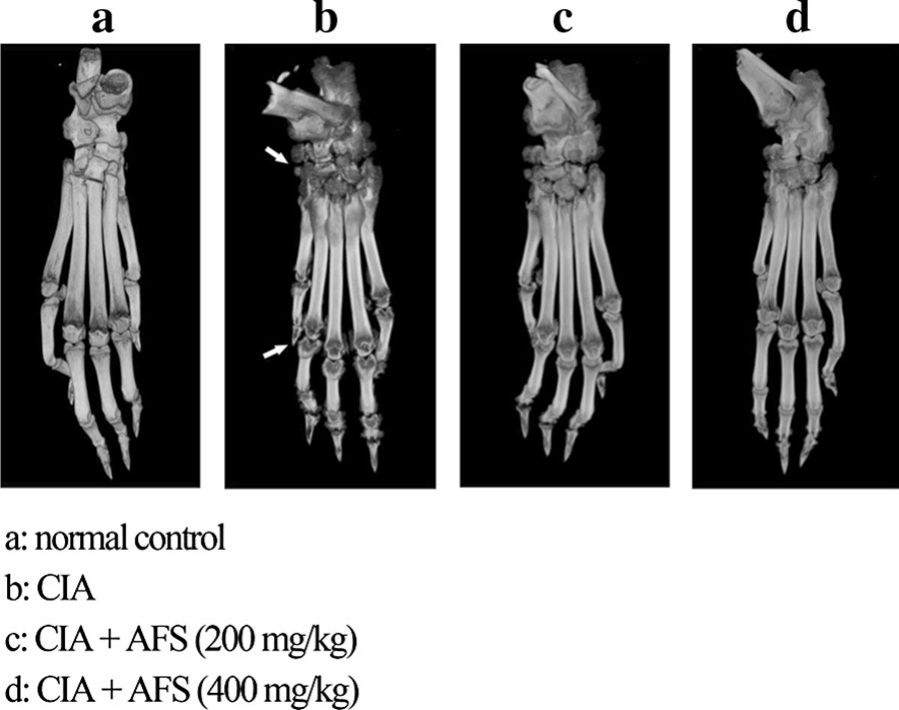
Effects of AFS on joint damage and bone structure in CIA. Representative radiographs obtained after 30 days of AFS treatment demonstrating remarkable amelioration of the articular destruction. *Arrows* indicated remarkable soft tissue swelling and large bone erosion.
